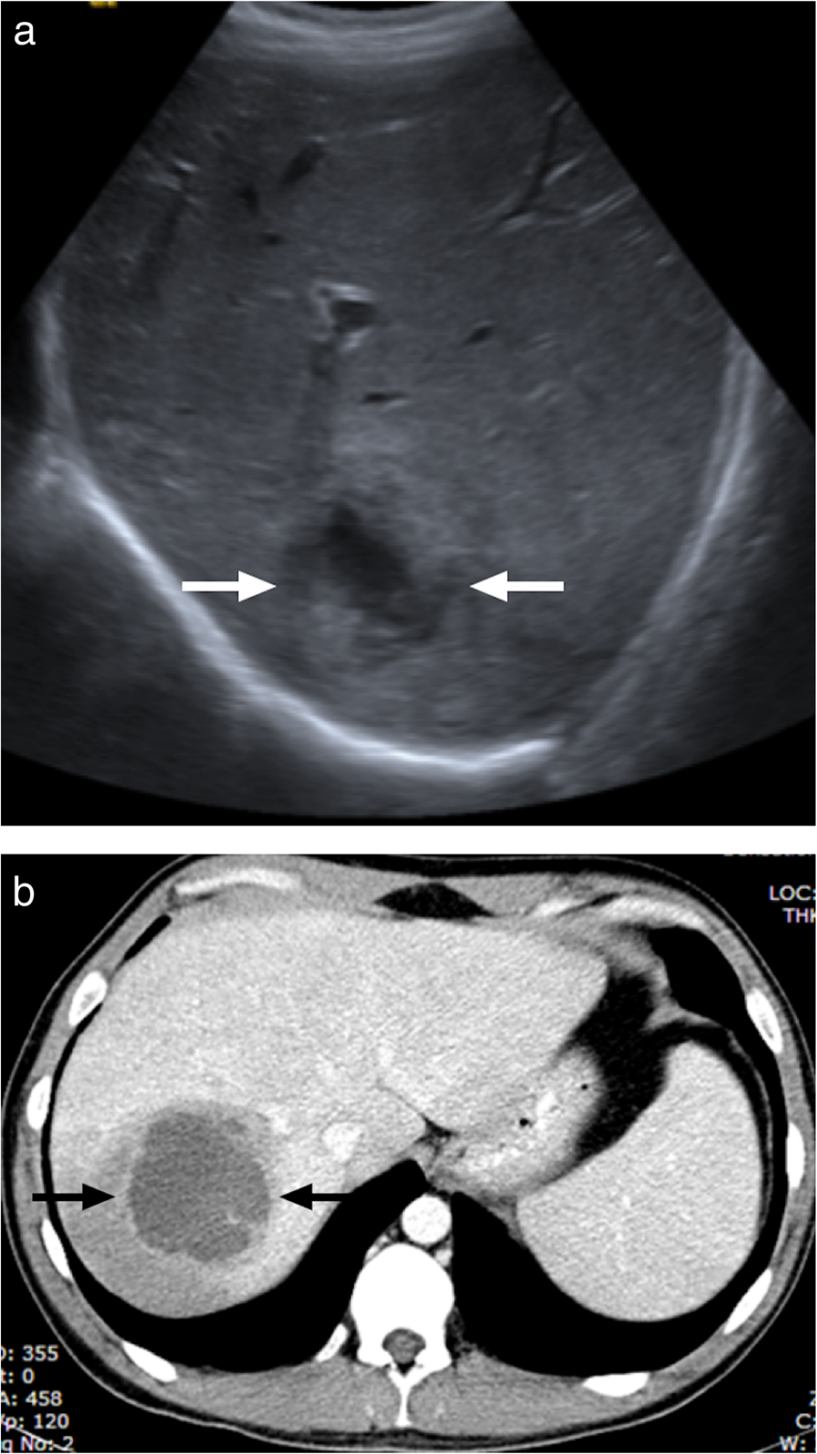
A 32-year-old male with no known medical history now presented with right upper quadrant pain and fever. His personal history was remarkable for a recent trip to India. a Sonographic study revealed semisolid mass located within the posterior segment of the right liver lobe. b Axial plane postcontrast CT image demonstrates thick-walled, centrally necrotic mass lesion (arrows) with peripheral hypodense area consistent with edema. Percutaneous sampling revealed amebic liver abscess
